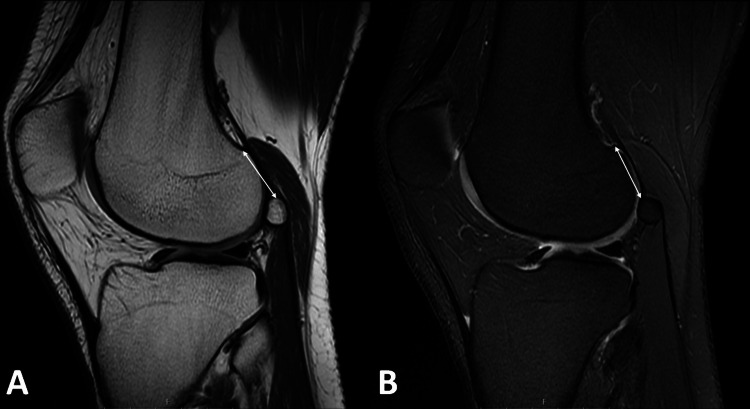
Sagittal proton density-weighted (A) and proton density fat-saturated (B) MRI views demonstrating the measurement of the distance between fabella to the insertion point of the lateral head of the gastrocnemius muscle (LG) using the double arrow.
